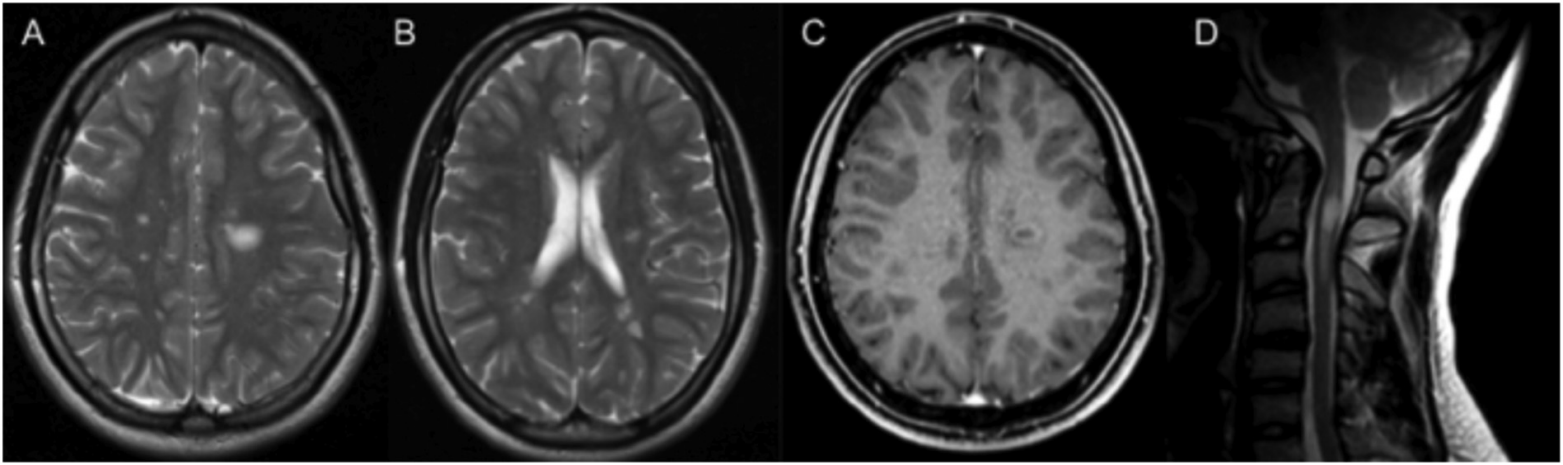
Brain and spinal MRI of the patient at the age of 17 (A) Note the disseminate white matter lesions on the axial T2-weighted sequence. (B) Lower section shows more asymmetrical and multifocal hyperintense lesions of white matter. (C) The large left white matter lesion shows and open-ring enhancement on the axial T1-weighted contrast sequence. (D) T2-weighted image of the cervical spine shows an hyperintense lesions at C2-C3 level.
